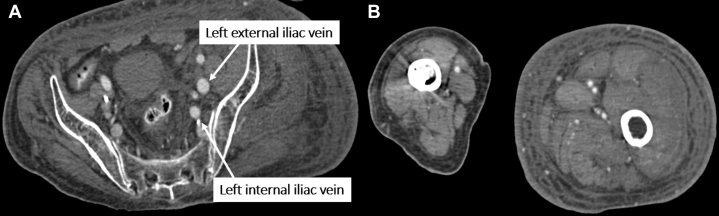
(A) CT angiography demonstrated arterial opacification of the left iliac veins (arrows), associated with swelling of the left lower limb (B). CT, computed tomography.
